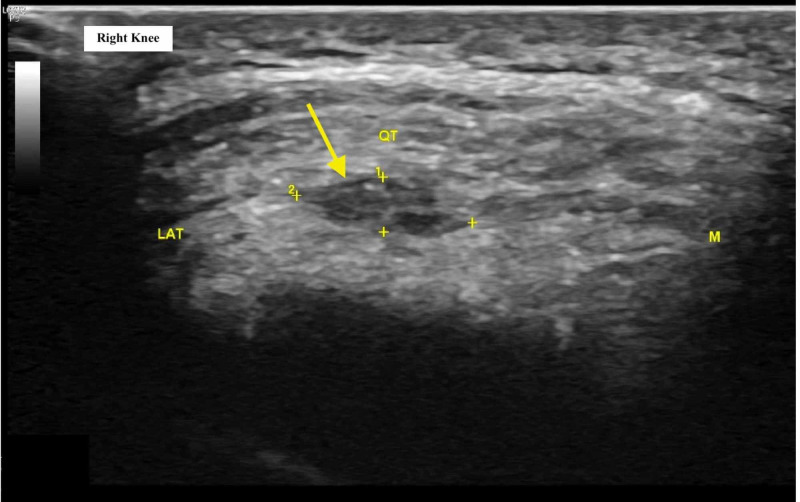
Short-axis ultrasound image of the right knee at initial visit demonstrating evidence of quadriceps tendinopathy, micro tears, calcific enthesopathy, and intrasubstance hypoechogenicity (yellow arrow) LAT: lateral anatomic orientation; M: medial anatomic orientation; QT: quadriceps tendon
